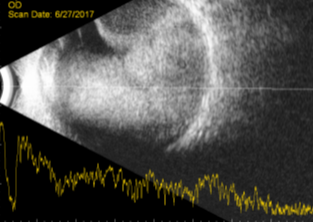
B-scan ultrasound of the left eye showing a multilobulated vitreous mass occupying practically the entire ocular globe with associated superior choroidal detachment
